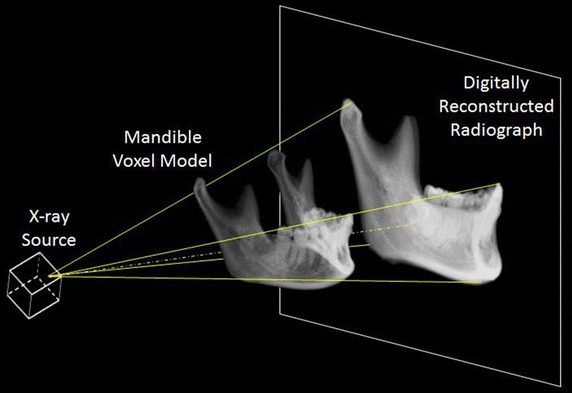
Generation of a synthetic cephalogram image of the mandible from the CBCT volume of the mandible using a digitally reconstructed radiography (DRR) technique. The radiography system was modeled as an ideal perspective projection of a point source X-ray through the bone onto the image plane. The CBCT volume of the mandible was positioned within the radiographic projection model with the principal axis of the projection defined as the line connecting the most prominent points on the medial surfaces of the bilateral condyles
